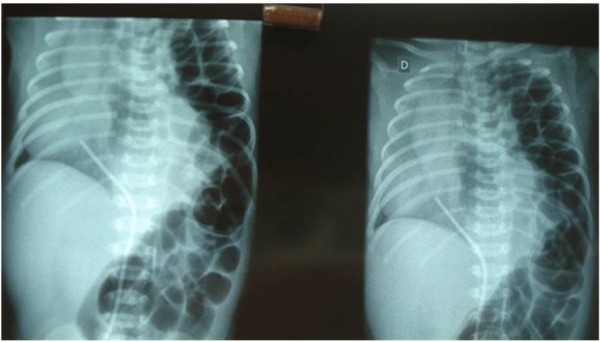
X-ray indicating diaphragmatic Bochdaleck.
